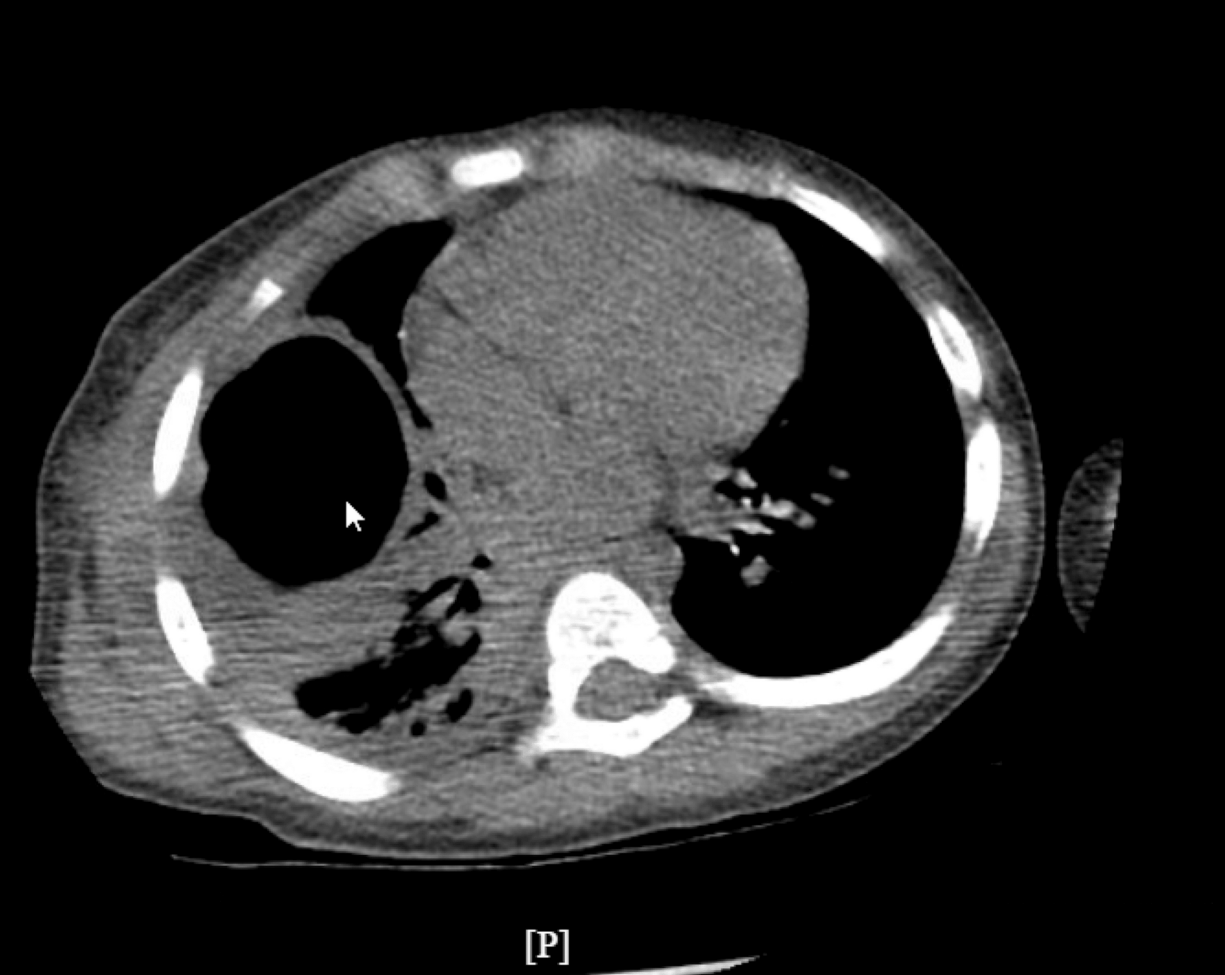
CT chest (transverse view), hospital day 1; large thick-walled cavity in the right upper lobe with surrounding smaller cavitary lesions.
